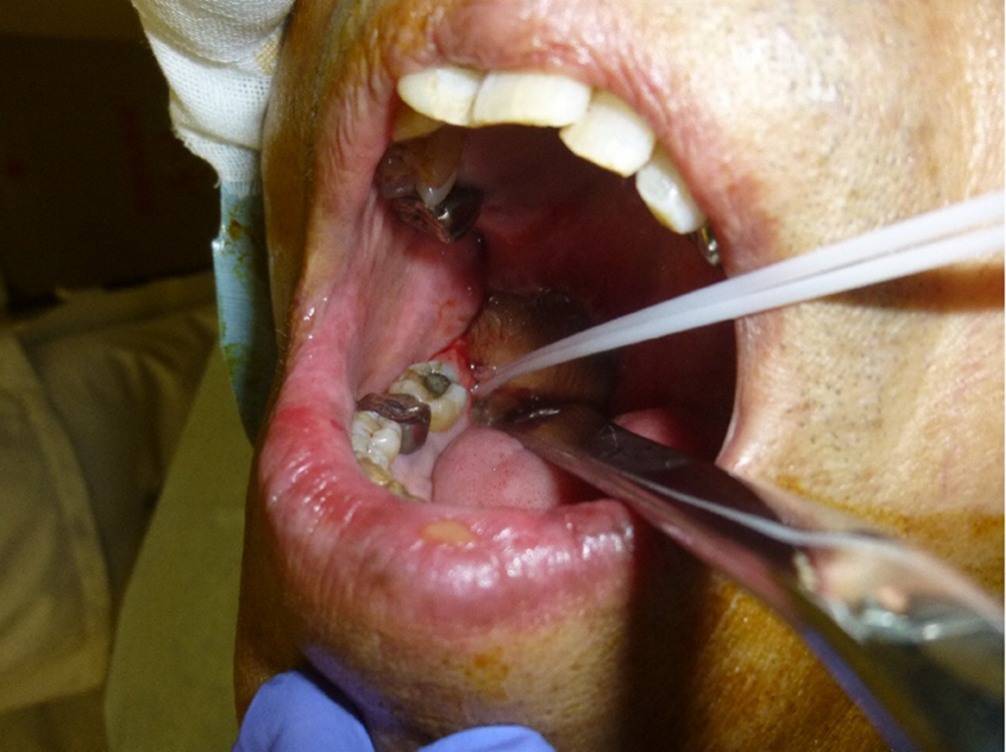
Under local anesthesia and sedation, two 5 French ProGUide^®^ plastic needles (Nucletron BV, Veenendaal, The Netherlands) were inserted transorally through the ALT flap. Depth of the needles were determined by CT image. ALT, anterolateral thigh.
